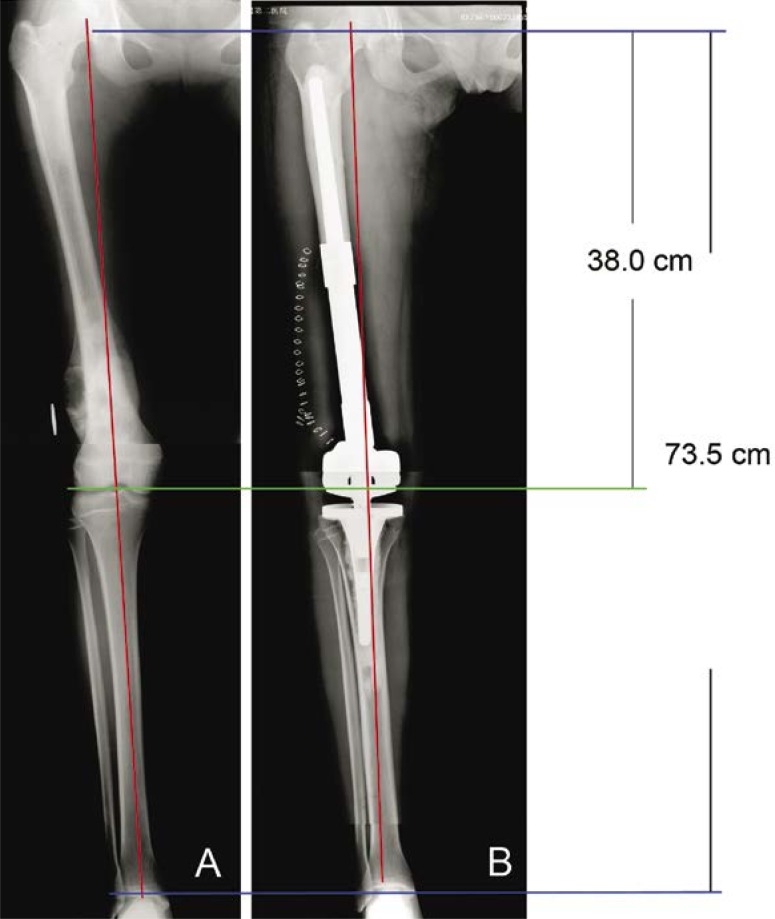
Postoperative assessment of prosthesis. A female, 19-year-old patient with osteosarcoma in the distal femur. Preoperative anterior-posterior plain film (A) and postoperative anterior-posterior plain film (B) reveal that the length and alignment were accurate after reconstruction. The red line showed the alignment of lower limb.
